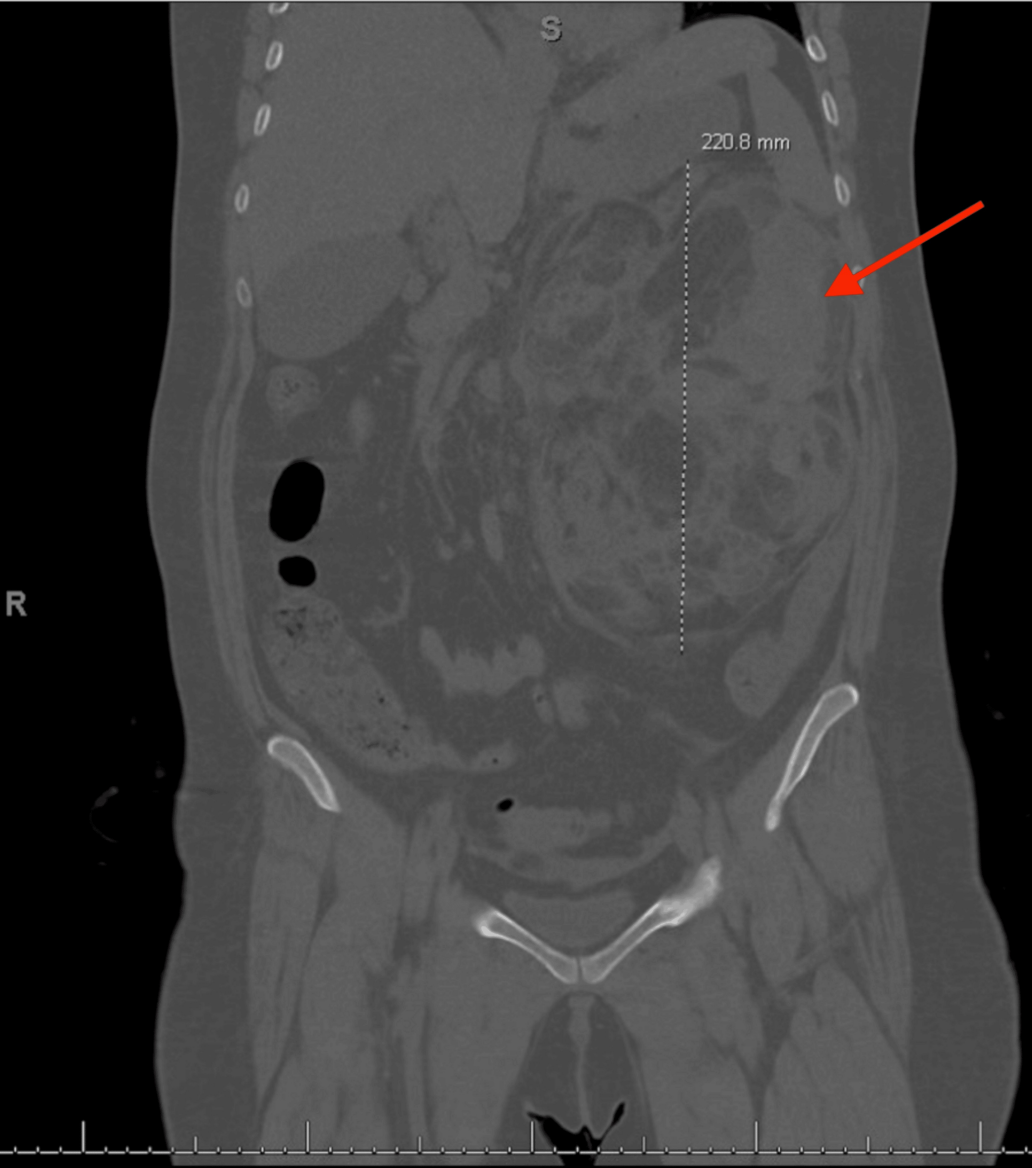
CT angiogram of the abdomen and pelvis: large left angiomyolipoma, measuring 12.8 x 12.8 x 21 cm with left perinephric hematoma in the coronal plane
